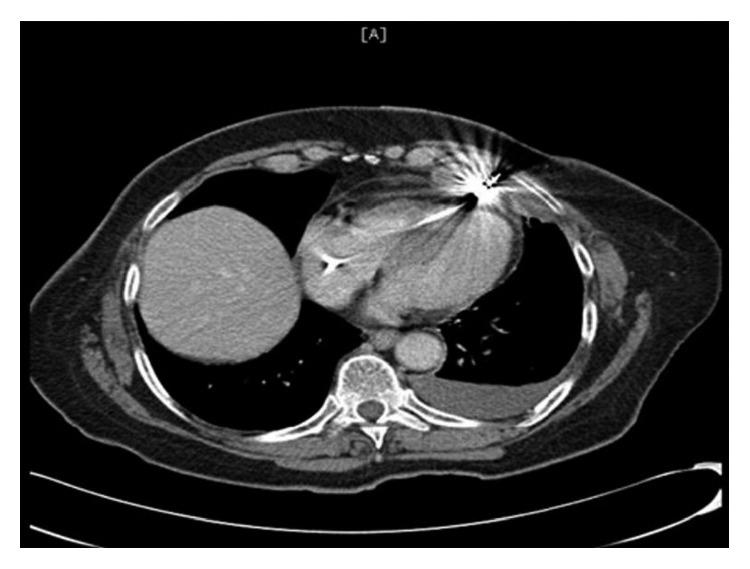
CT scan showing the tip of the right ventricular lead penetrating the anterior wall of the right.
